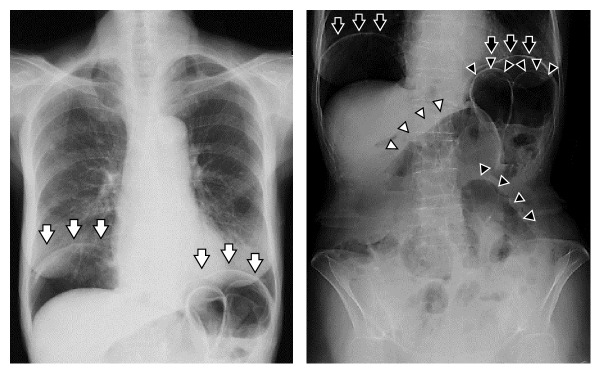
A chest radiograph demonstrating pneumoperitoneum in the subdiaphragmatic regions bilaterally (arrows). A plain abdominal radiograph showing several dilated loops of small intestine (arrowheads).
